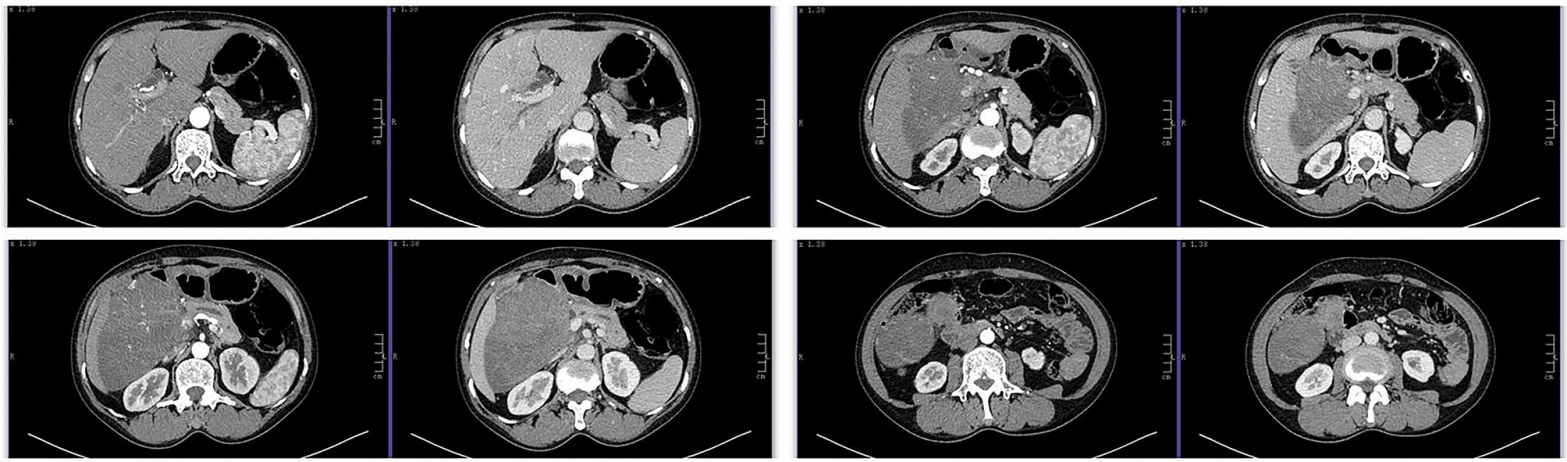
Computed tomography (CT) scan showed a retroperitoneal soft tissue density mass, 11 cm in size, which is closely related to the liver, first and third hepatic portals, portal vein, hepatic artery, and pancreas.
